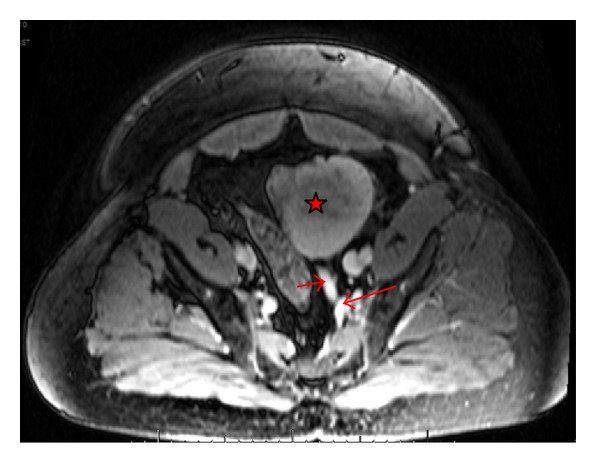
Venous Phase T1-weighted MRI image (transverse view) showing anomalous venous drainage from ectopic left kidney and mass (star). Left renal vein (short arrow) draining into left internal iliac vein (long arrow).
